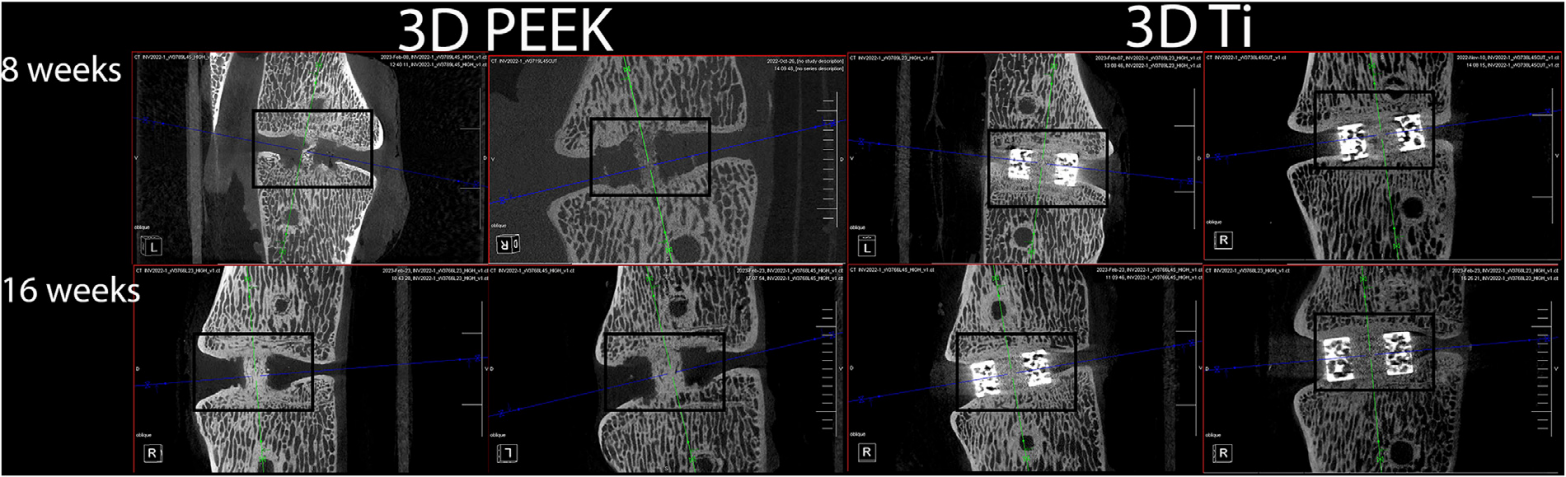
Examples of sagittal micro-computed tomography images (38 microns) for 3DPEEK and 3DTi at 8 and 16 weeks. The 3DPEEK cages are not visible while the progression in bone fusion within the aperture as well as osseointegration into the cage can be seen in the microcomputed tomography scans. The 3DTi cages were noted along with image artifact that precluded any definitive determination of bone within the aperture as well as within the porous regions of the 3DTi cage.
